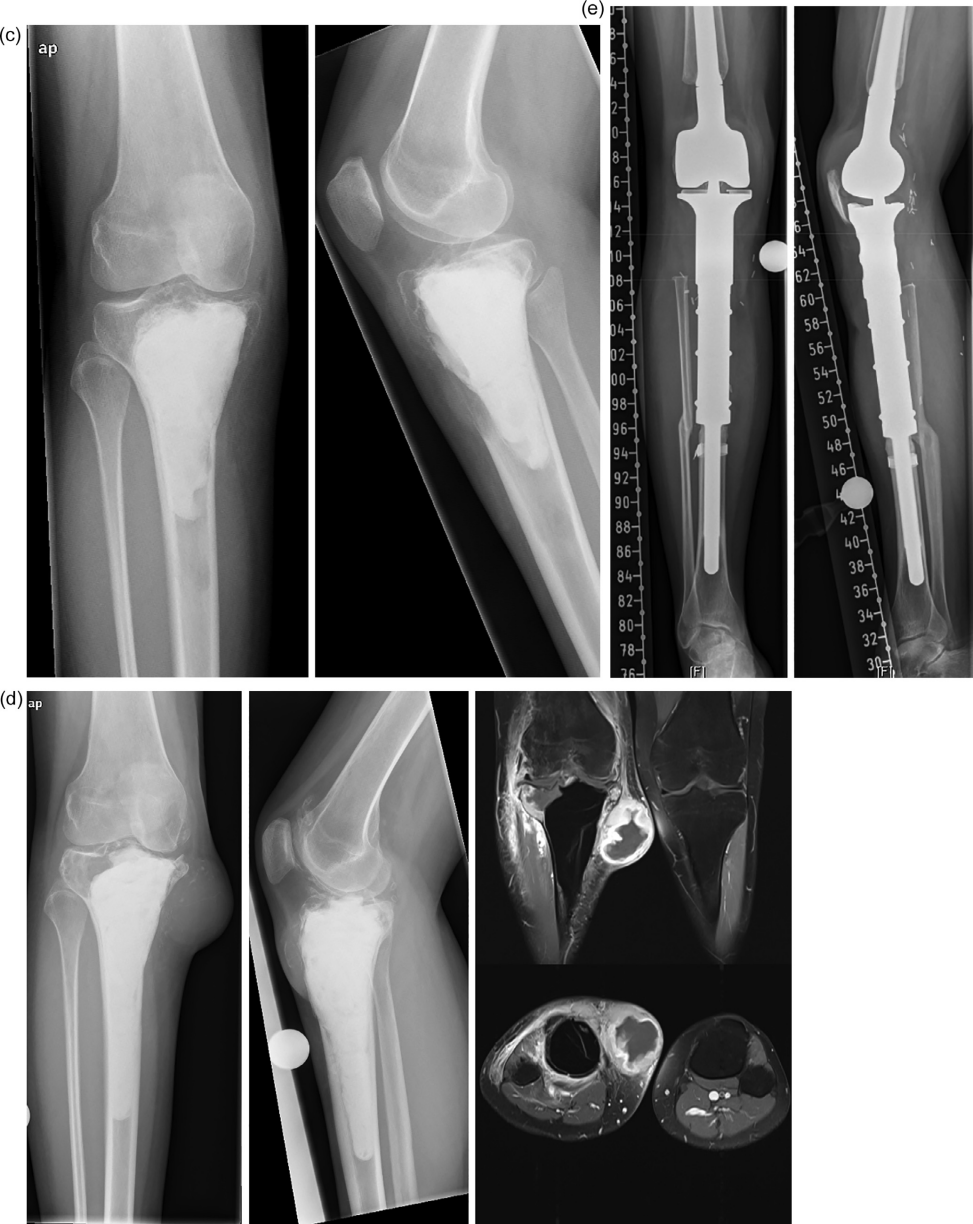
a-e: case of a 39-year-old female patient with GCTB of the proximal tibia: 3a: radiographs (left and middle) and MRI-scan (middle and right) of the proximal tibia showing an osteolysis; 3b: postoperative radiograph after intralesional curettage and defect reconstruction with bone cement; 3c radiographs of first local recurrence 18 months after surgery; 3d: radiographs (left and middle) and MRI-scan (middle and right) of second local recurrence 9 months after second curettage; 3e: radiographs of a modular tumor endoprosthesis which had to be implanted due to massive bone defect.
